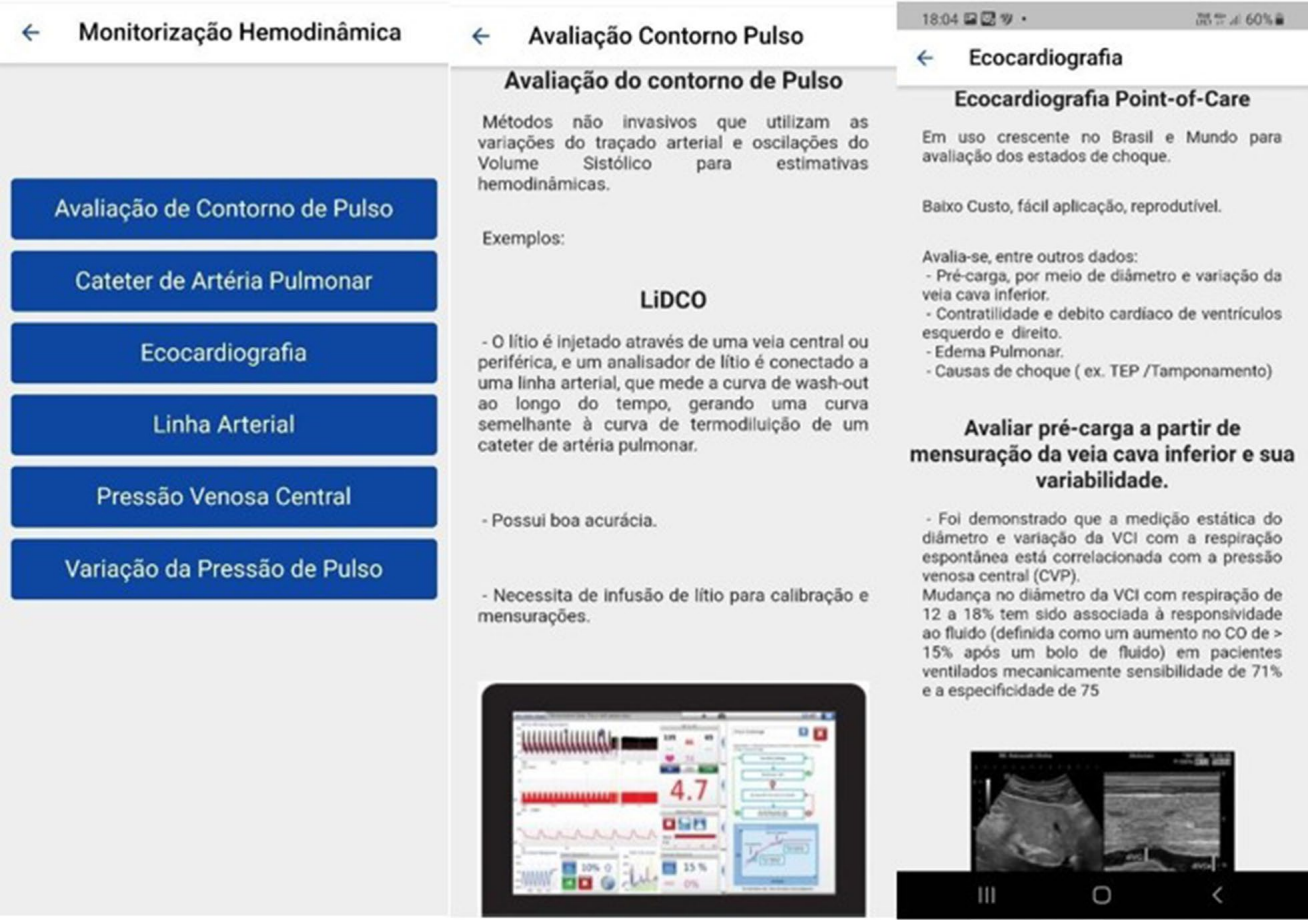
Example of the application educational tool. In the image on the left, list of tool options for hemodynamic monitoring: Pulse contour analysis, pulmonary artery catheter, echocardiography, arterial line, central venous pressure, pulse pressure variation. At the Center, example of the pulse contour evaluation interface. Right image, example of the text and figures from ultrasound use
